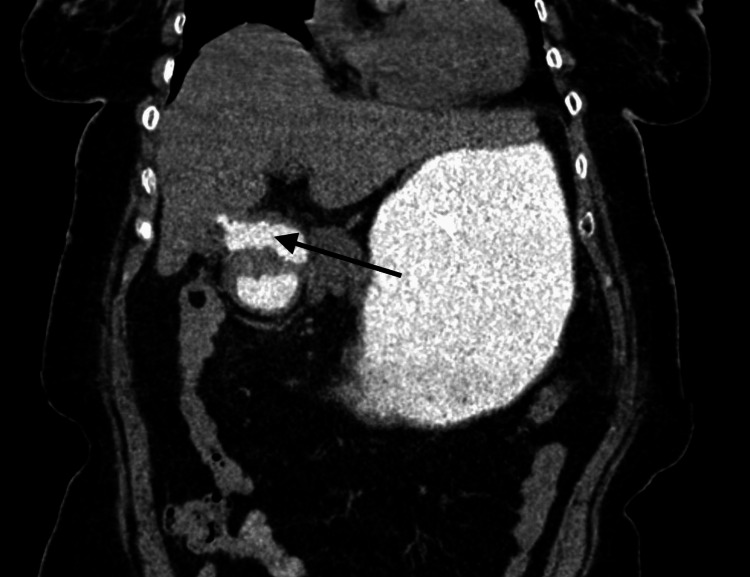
CT scan showing cholecystoduodenal fistula (black arrow)
